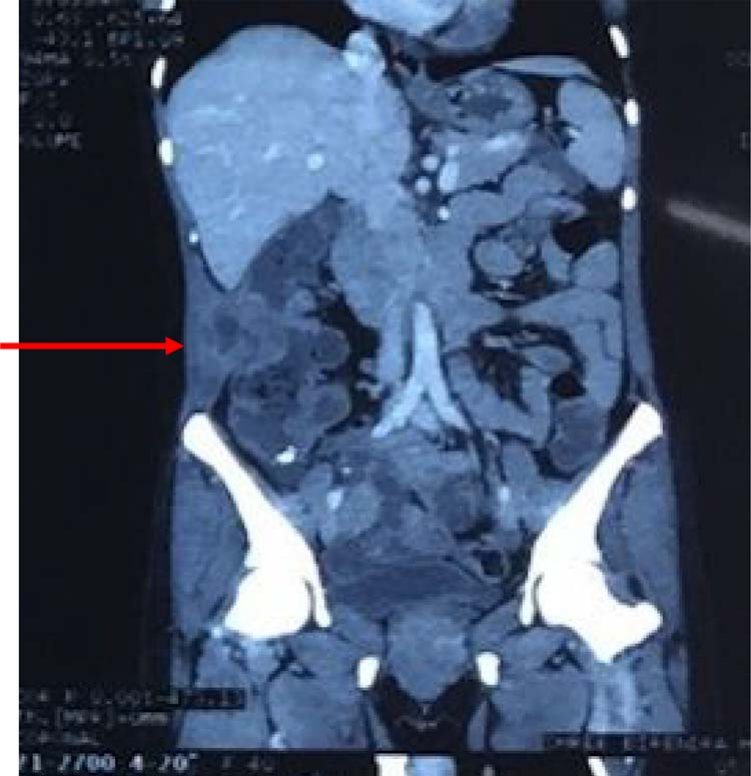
Coronal reconstruction of CT shows mass extending from colon and invading into anterior abdominal wall. Arrows indicate the point of exit of mass through the abdominal wall musculature.
